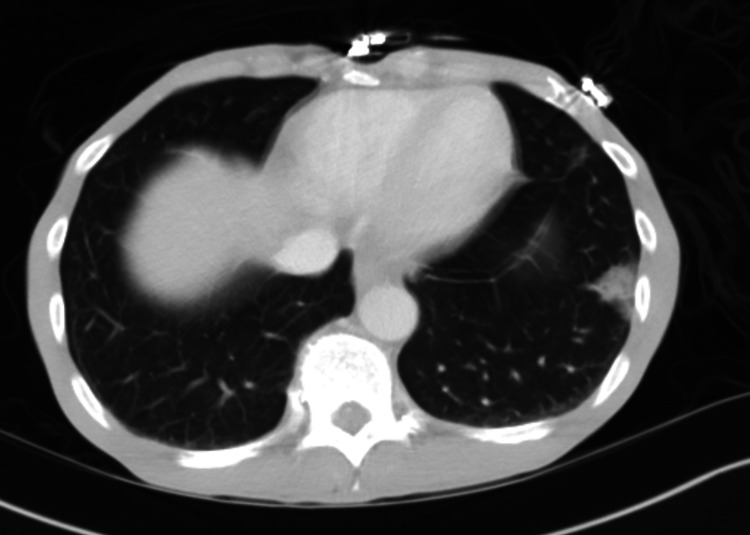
CT abdomen shows a peripheral ground-glass opacity involving the left lower lobe of the lung
